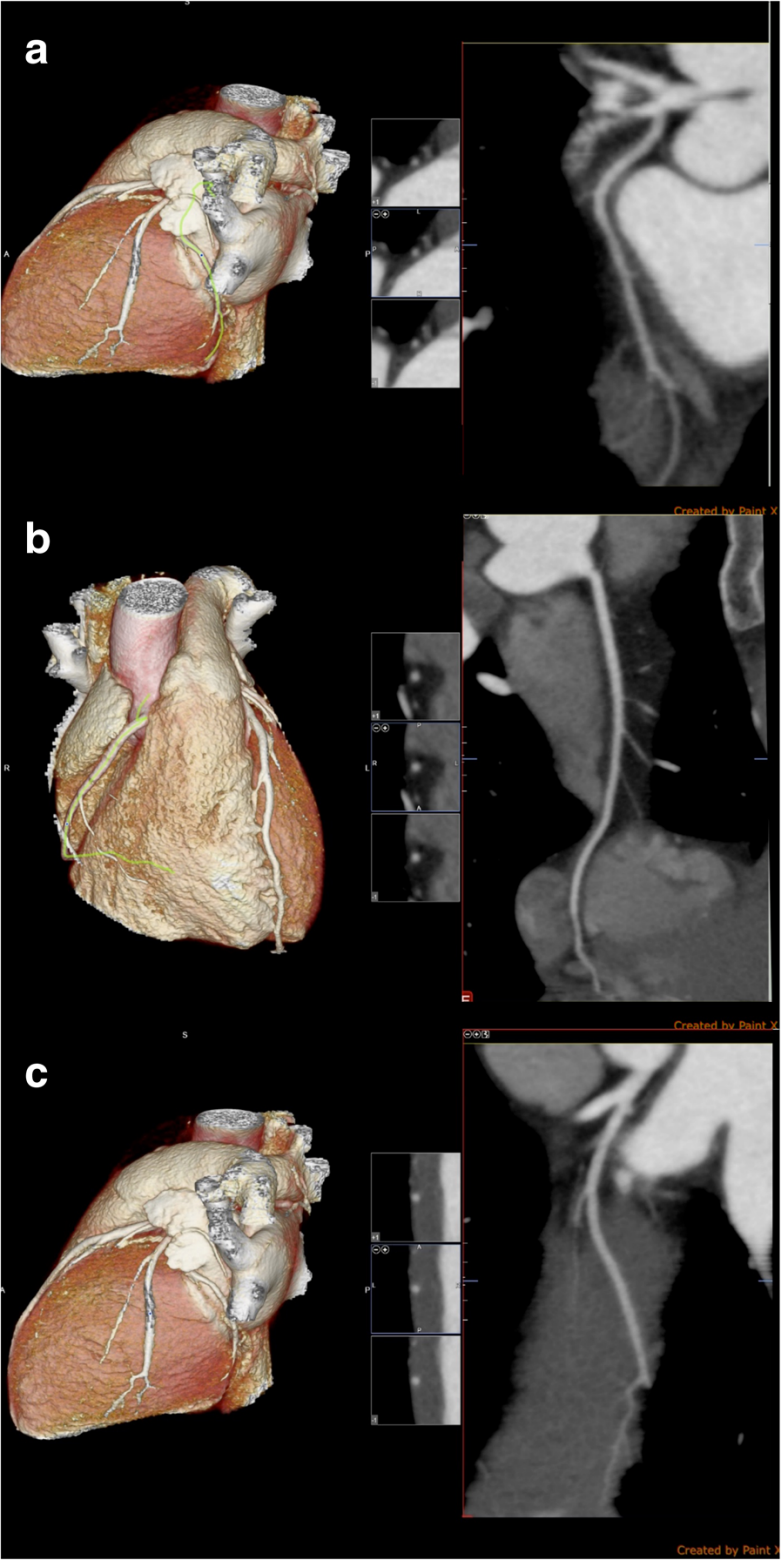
Normal CT coronary angiogram. a = Normal left circumflex artery. b = Normal Right coronary artery and posterior descending artery. c = Normal Ramus intermedius artery
